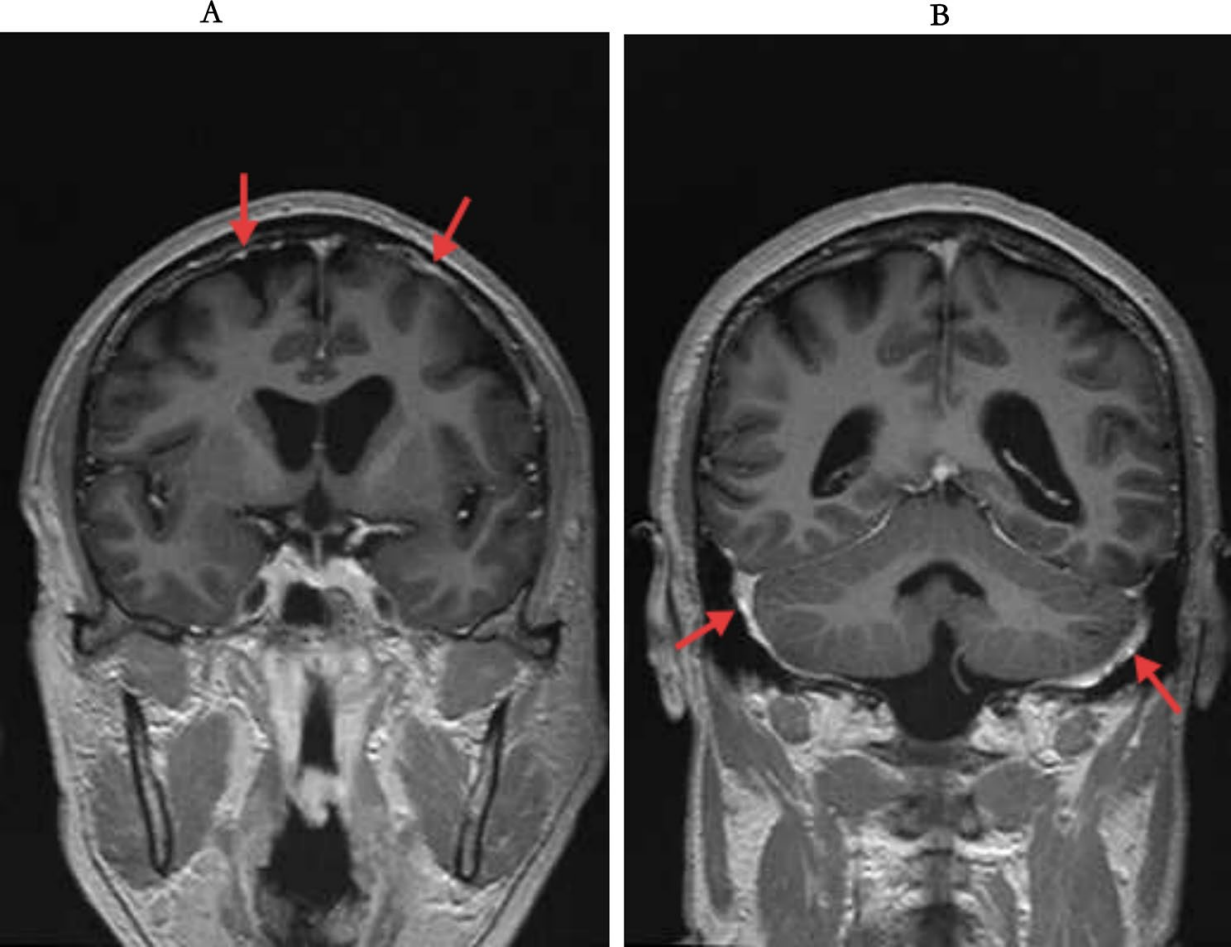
Brain MRI findings of leptomeningeal metastases on May 6, 2025. (A) Axial post-contrast T2-FLAIR image demonstrates characteristic linear leptomeningeal enhancement along the cerebral sulci; (B) Linear enhancement is also seen along the cerebellar sulci, with associated subcortical patchy hyperintensities
